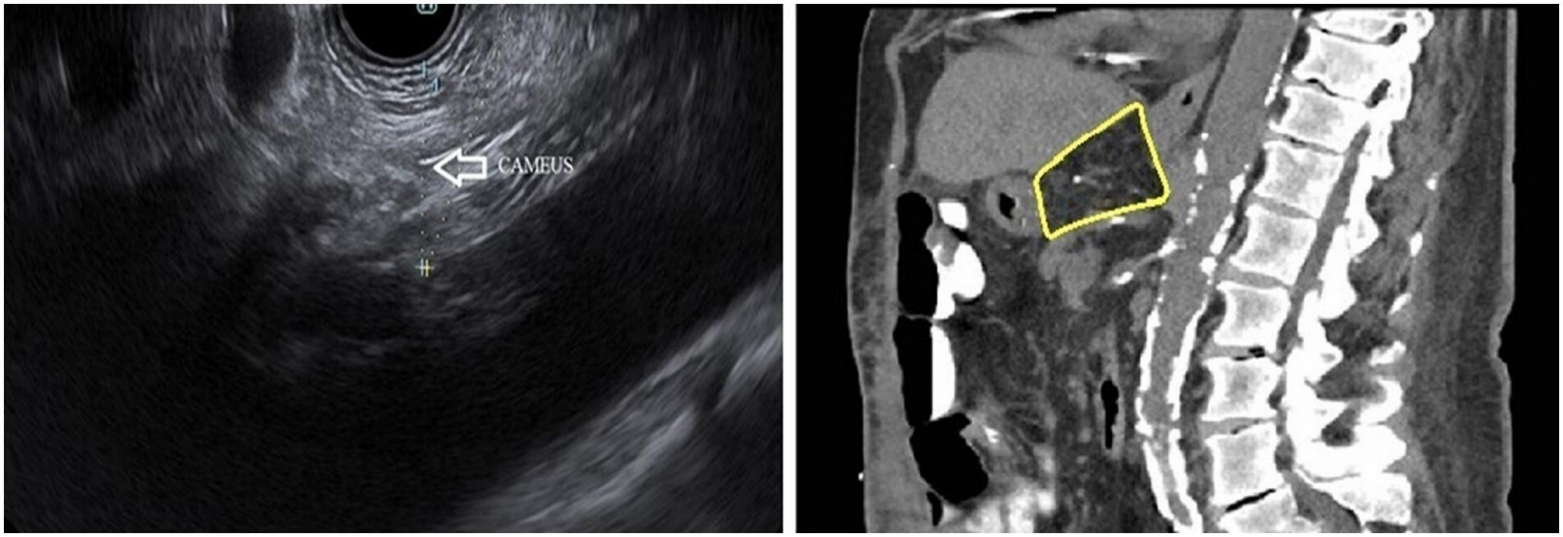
Endoscopic ultrasound estimation of celiac artery mesenteric fat thickness (left) and computed tomography scan correlate of celiac artery mesenteric fat thickness (right). CAMEUS = celiac artery mesenteric fat measurement with endosonography.
